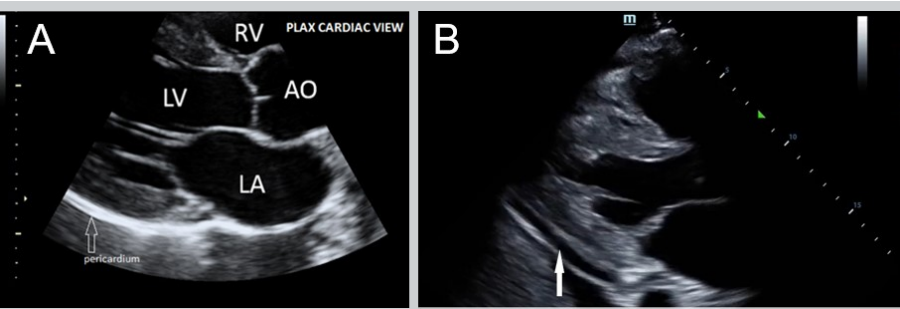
A) Focused cardiac ultrasound (FoCUS). Parasternal long axis view (PLAX) showing different chambers and aorta. B) Focused Cardiac Ultrasound. PLAX view showing pericardial effusion (white arrow).
